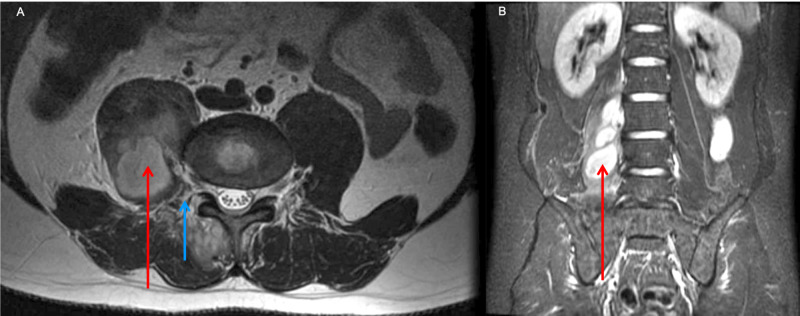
(A) Axial T2 MRI image showing the psoas muscle (red arrow) and facet joint lesion spreading to multifidus muscle (blue arrow). (B) Coronal T1 MRI post gadolinium showing extensive psoas muscle abscess (red arrow).
